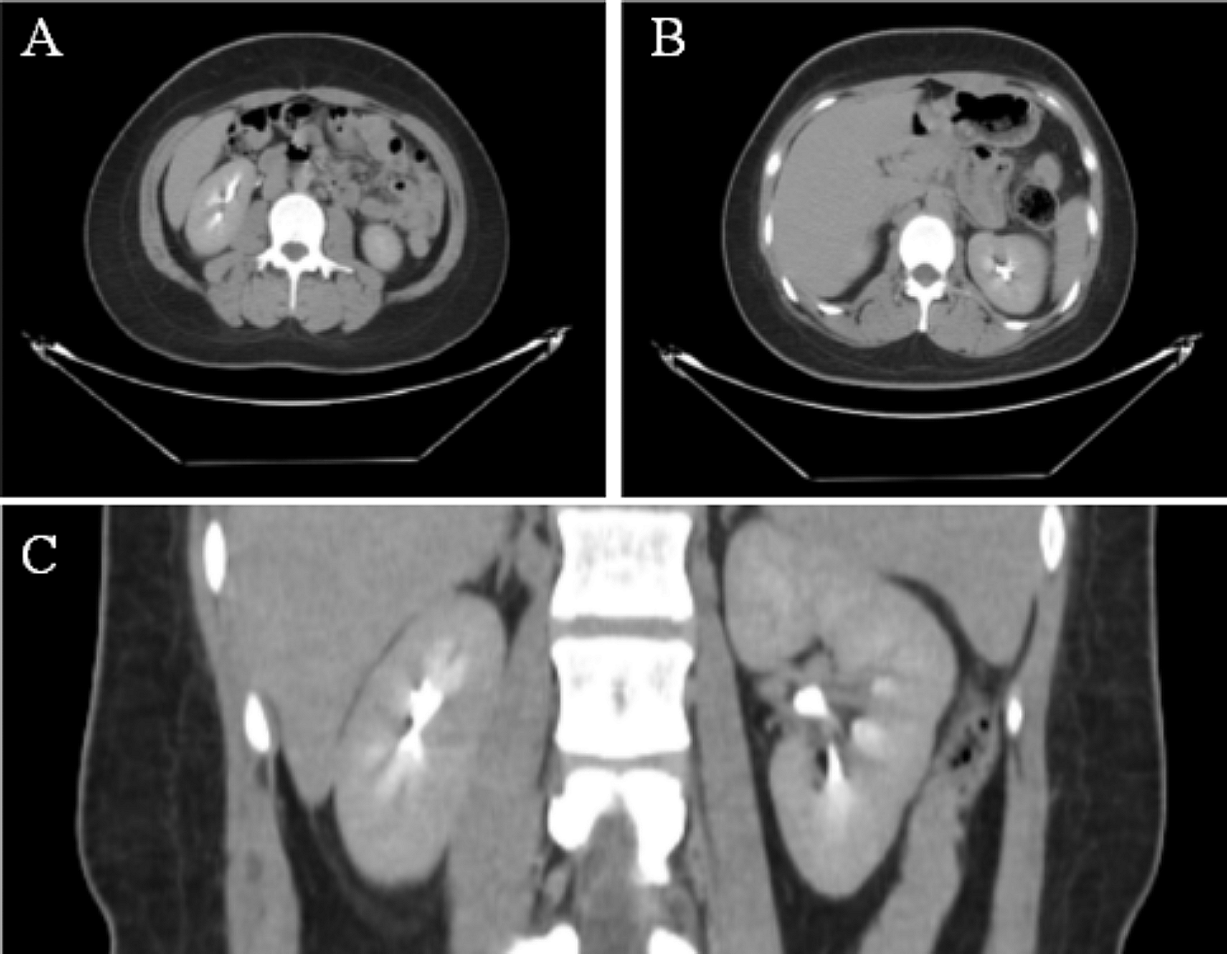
Enhancement CT of urinary system. Increased diffuse density in the medullary area of both kidneys with small stones. (A, B) Cross-section. (C) Coronal plane
